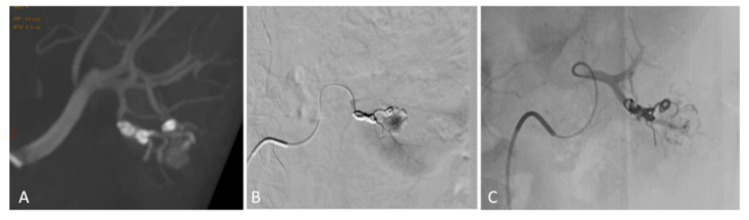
Intra-procedural CBCT (A) and arteriogram (B) respectively confirmed blood penetration through the coils. One millimeter of NCBA (1:1) was selectively injected to stop the flow behind the coils. Final angiogram, after embolization with one millimeter of NCBA (1:1), showed complete exclusion of PSA (C).
